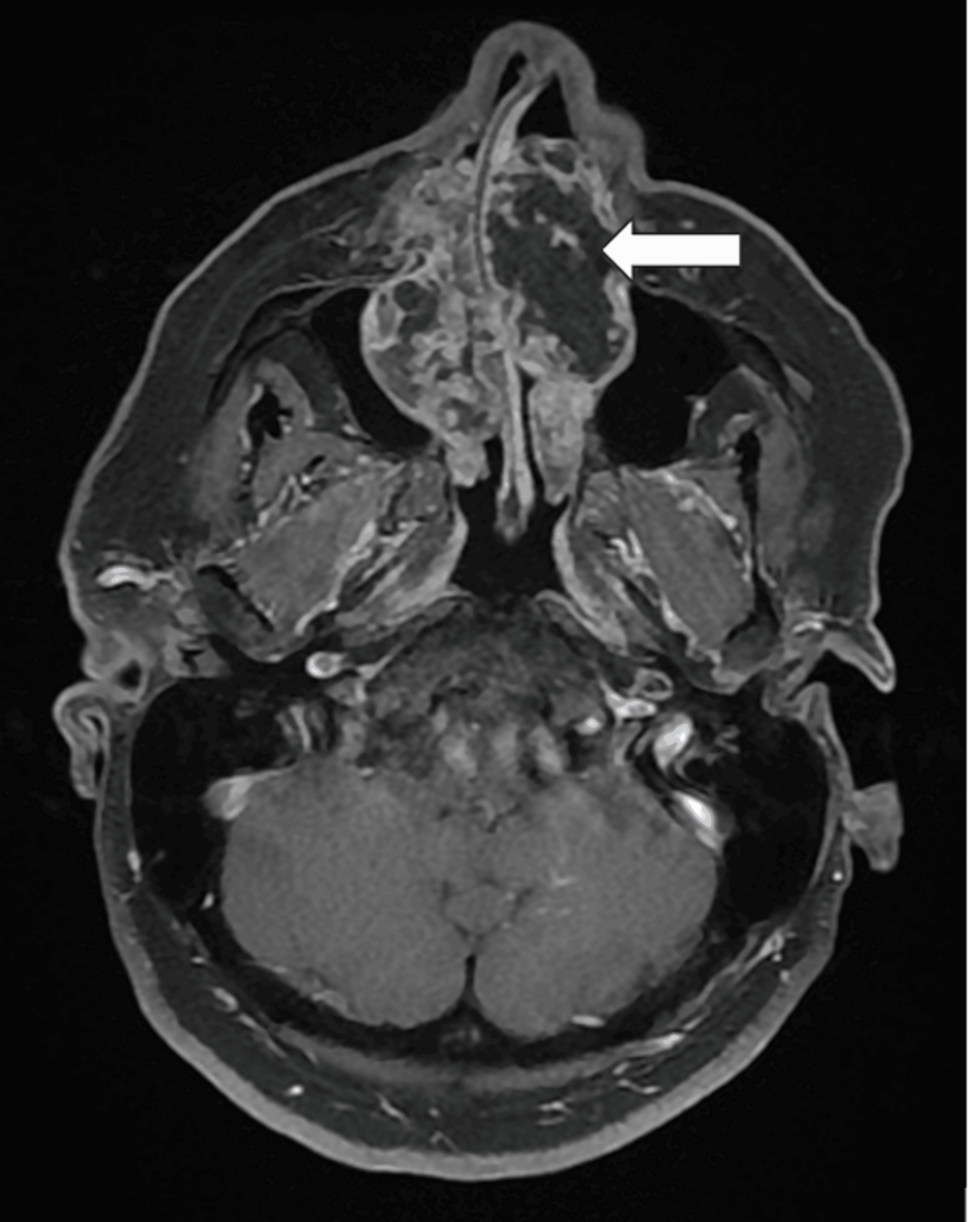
Axial post-contrast T1-weighted fat-suppressed MRI The image demonstrates postoperative changes after subtotal maxillectomy (red arrow) with restoration of the sinonasal anatomy and preservation of the orbital floor. No residual or recurrent enhancing lesion is seen MRI: magnetic resonance imaging
